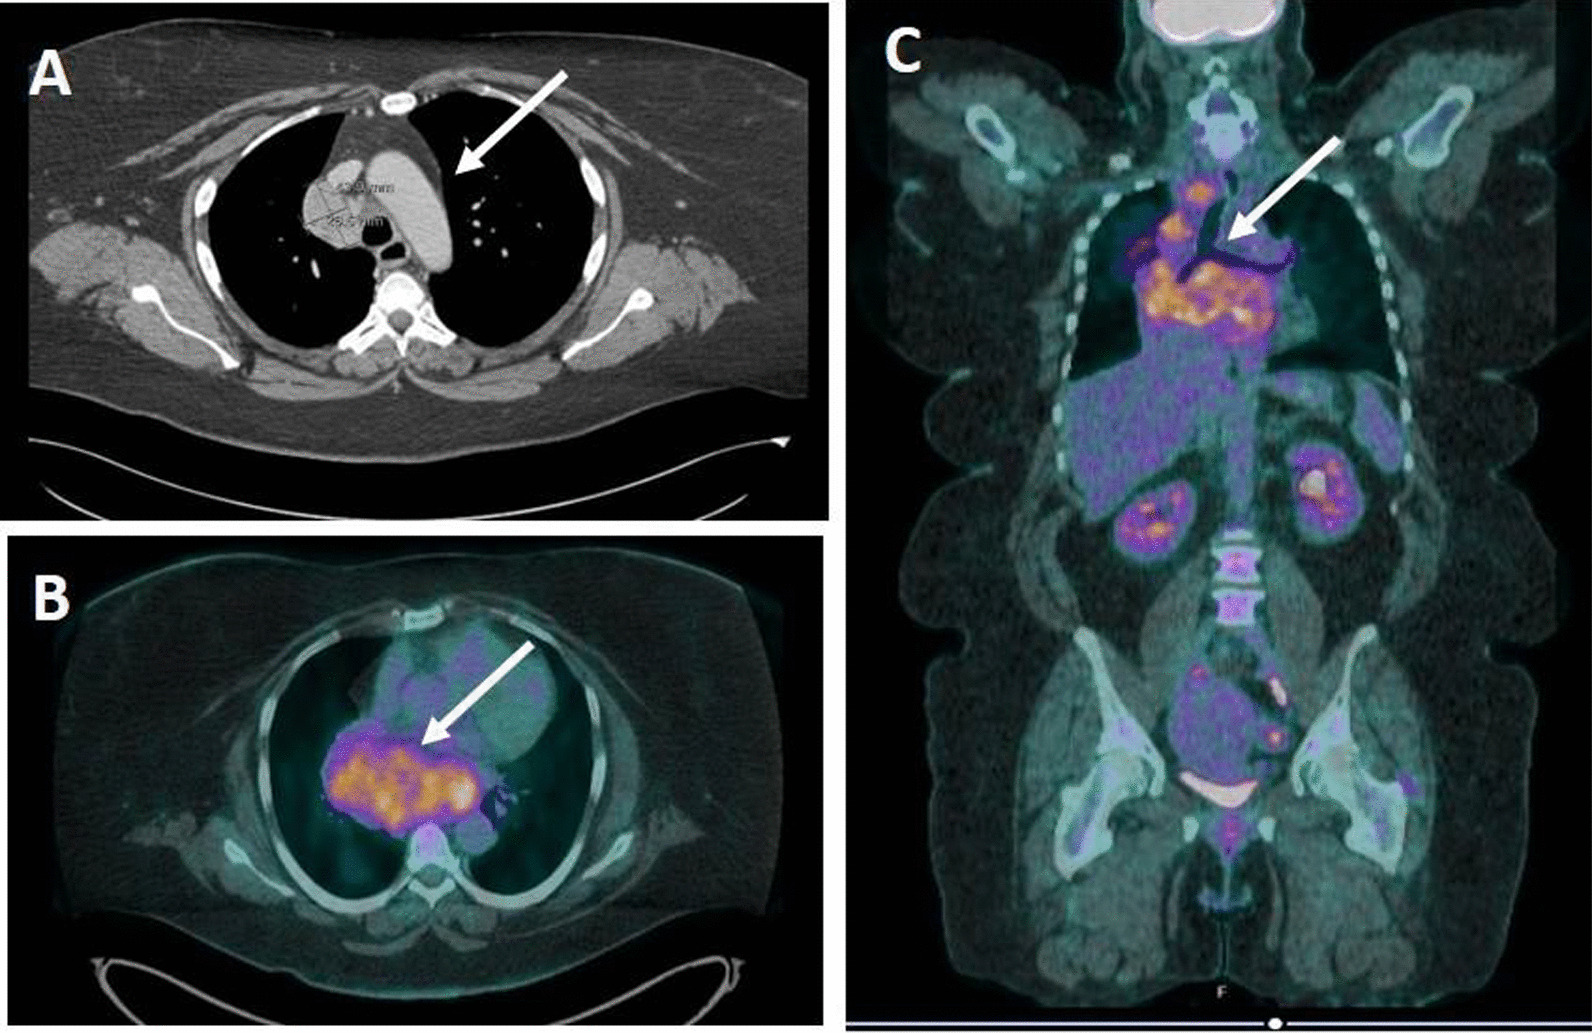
Imaging of the mediastinum mass. A Transverse computed tomography image demonstrating the mass (arrow). B, C Transverse and coronal plane positron emission tomography scan images highlighting the mediastinal mass obstructing the esophagus (arrows)
